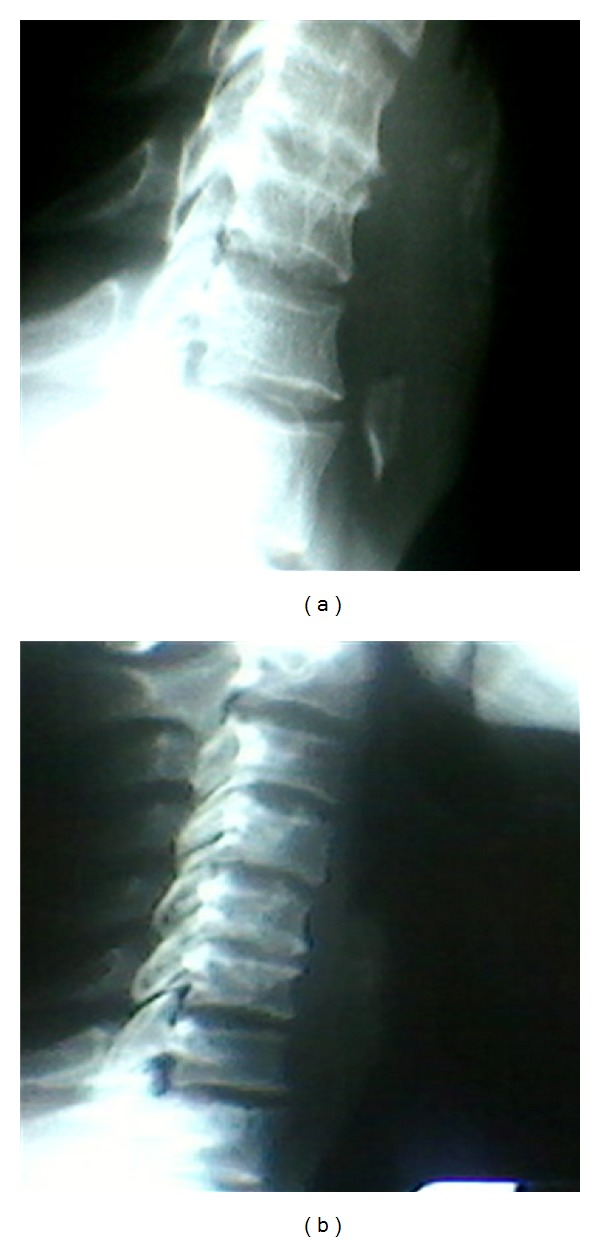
Lateral neck radiographic image of a chicken bone embedded in the retropharyngeal space (a) and after endoscopic removal of foreign body (b).
